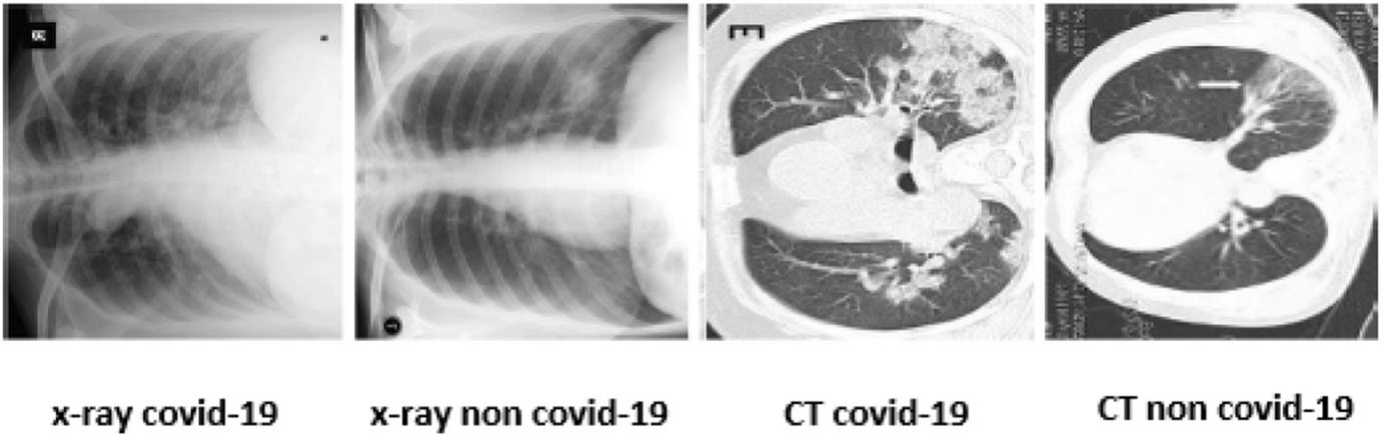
Main features of COVID-19 for both CT and X-ray images
